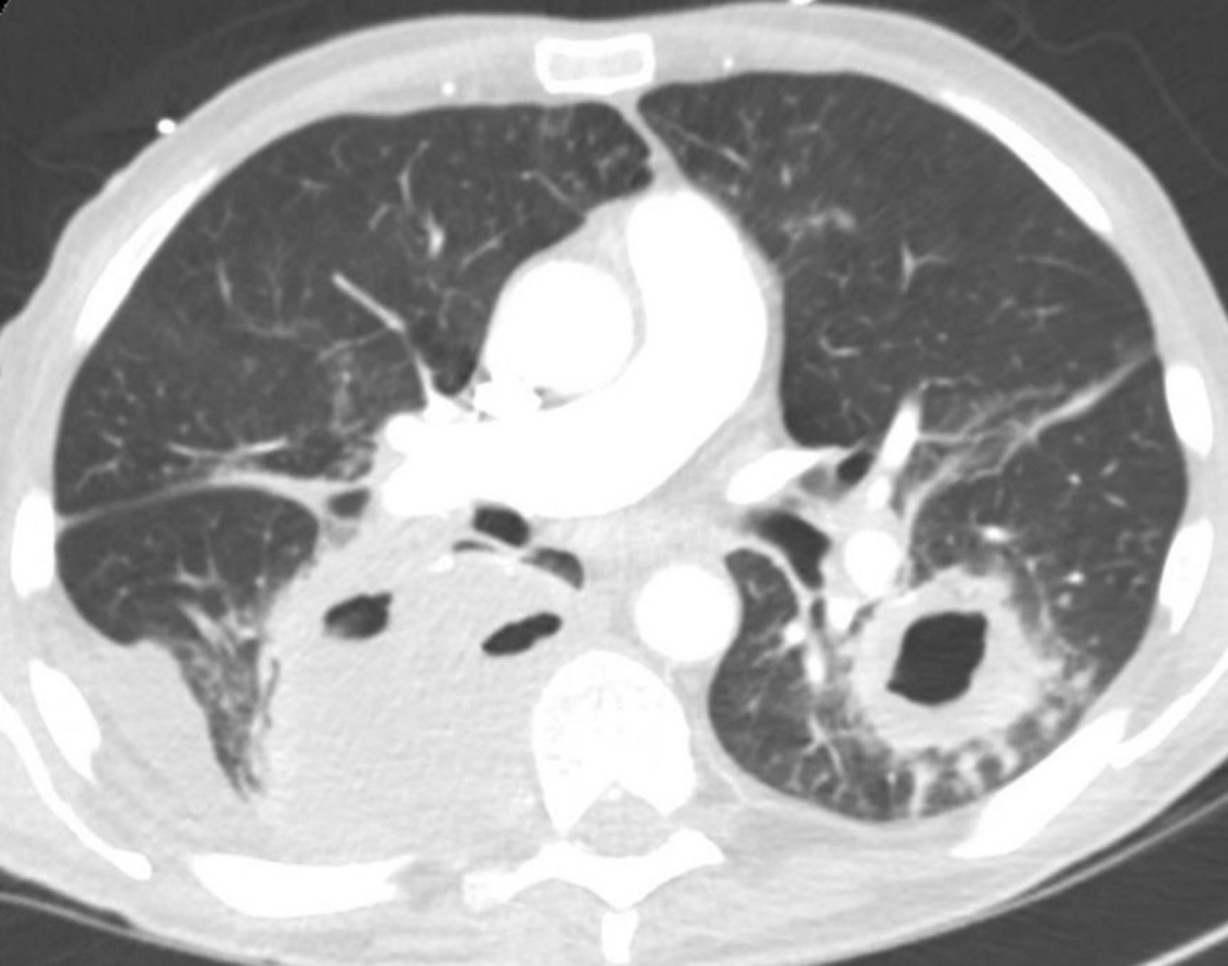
CT angiogram of the chest in transverse view revealing large bilateral lung abscesses.
